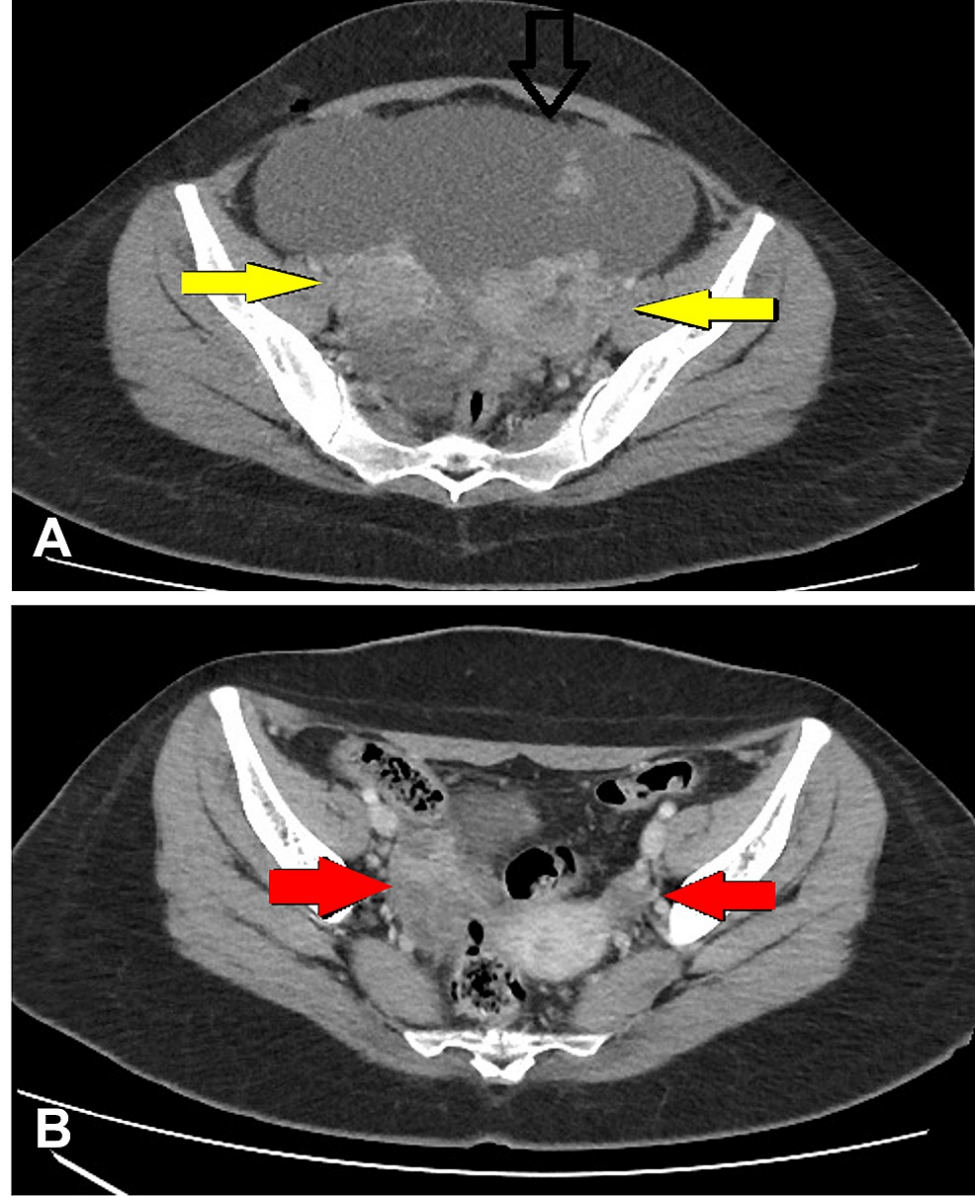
(A) Axial contrast-enhanced CT scan through the pelvis. An interim scan two months later shows disease progression with interval development of bilateral adnexal masses, ascites and peritoneal nodules (yellow arrows). (B) Axial contrast-enhanced CT scan through the pelvis. Interval treatment response status post neoadjuvant chemotherapy. There is an interval decrease in the size of adnexal masses (left more than right) and ascites (red arrows).
